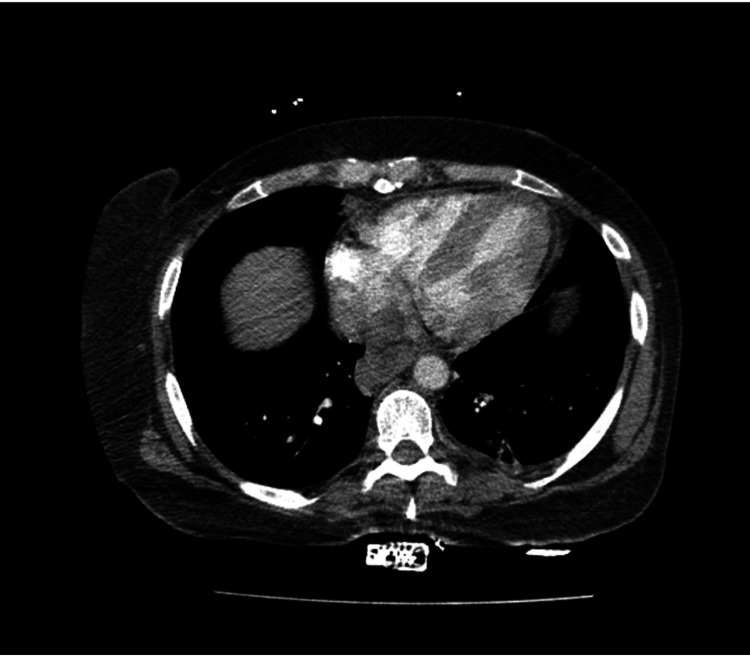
CTA of the chest showing left basilar lung infiltrate with associated minimal left pleural effusion CTA, computed tomography angiography
